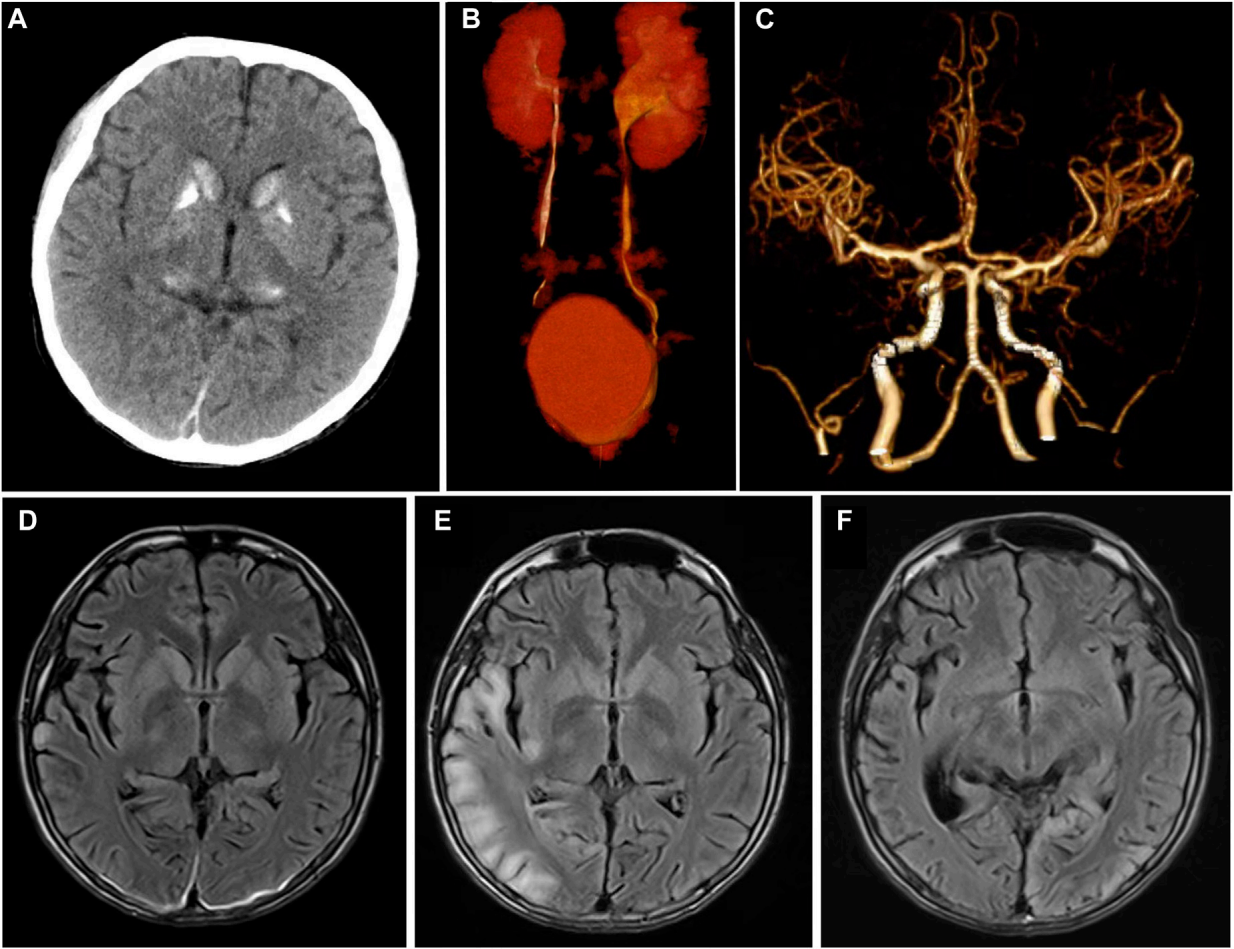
Imaging characteristics at admission and follow-up of this patient. (A) Symmetrical calcification of bilateral basal ganglia and thalamus was showed in head CT at admission; (B) CT urography showed that the left ureteropelvic junction was narrow with left hydronephrosis; (C) No abnormality in the head CTA. (D–F) Showed the cranial MRI T2flair characteristics of this patient at admission, after 1 month and 6 months of follow-up. After admission, only the right occipital cortex showed laminated high signal (D). One month later, the patient showed typical high signal intensity area in the right occipital lobe (E). Six months later, the right occipital lobe lesion disappeared and transferred to the left occipital cortex (F).
